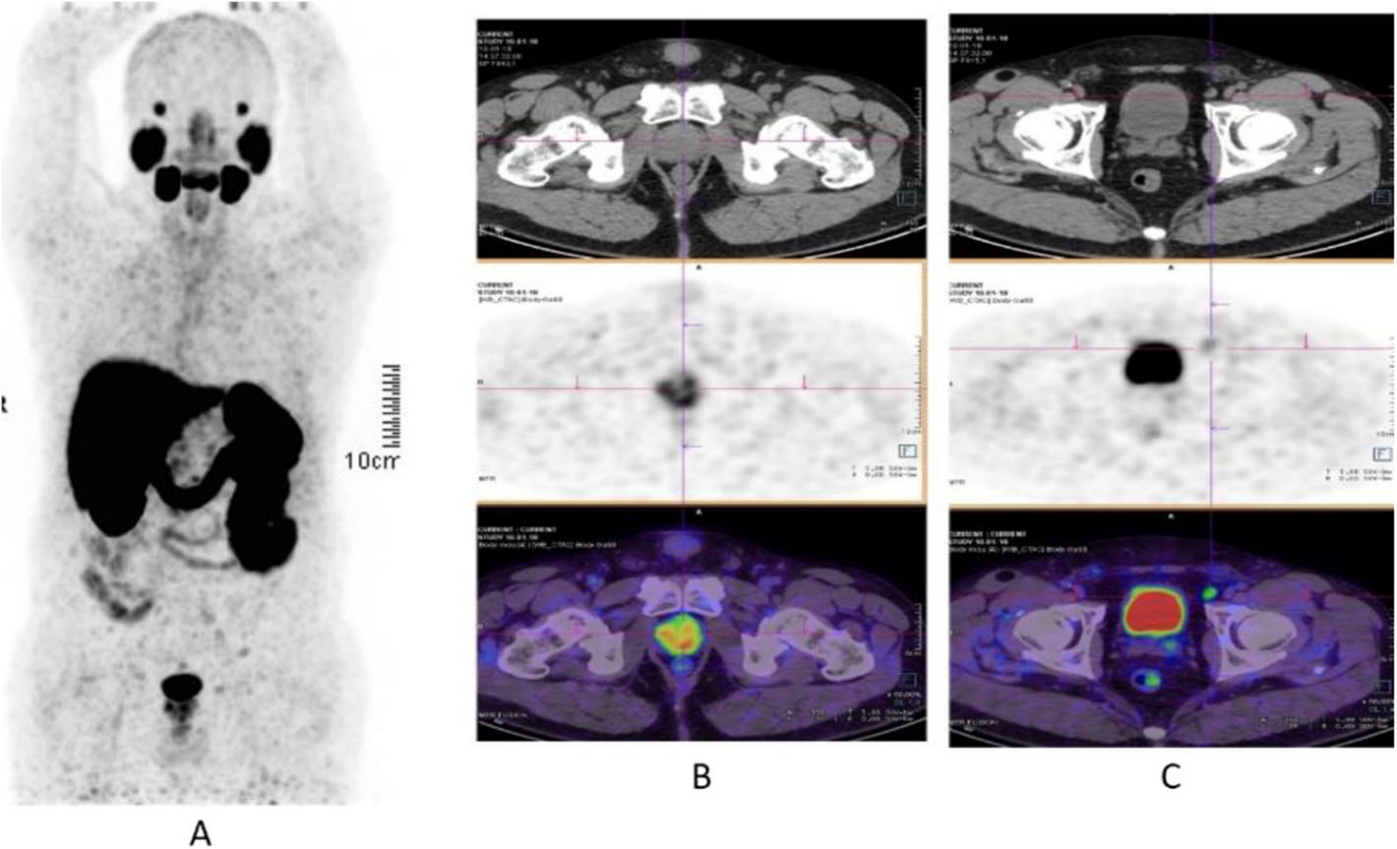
68Ga-PSMA, prostate cancer, initial staging. *Clinical history*: 58 y.o. man with prostate cancer, Gleason score 3 + 4. Suspicious rib lesion on bone SPECT/CT. *PET/CT findings*: increased uptake in prostate tumour. No abnormal findings in the skeleton. Faint uptake in inguinal and external iliac nodes, with abnormal appearance on CT, corresponding to inflammation. a MIP; b, c fused images
